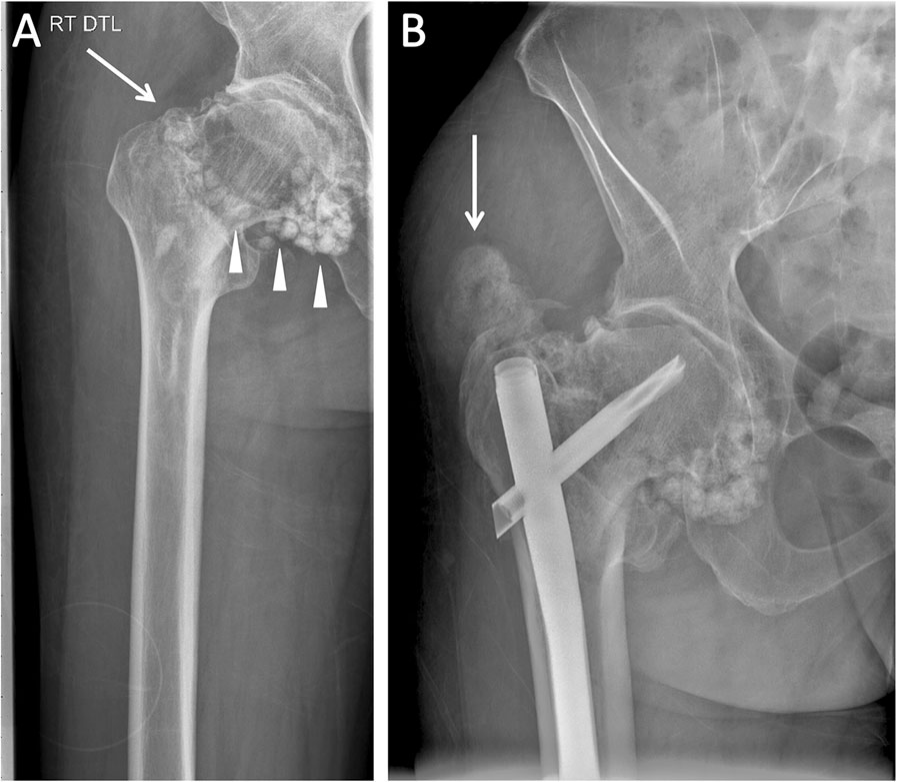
X-Ray imaging of the right hip: (a) Intertrochanteric fracture noted by arrow. There is longstanding osteochondromatosis at the right femoral head [arrowheads]. (b) X-ray of the femur 13 weeks after surgery show post-surgical hardware and demonstrate Grade B HO at the left femoral head [arrow]
